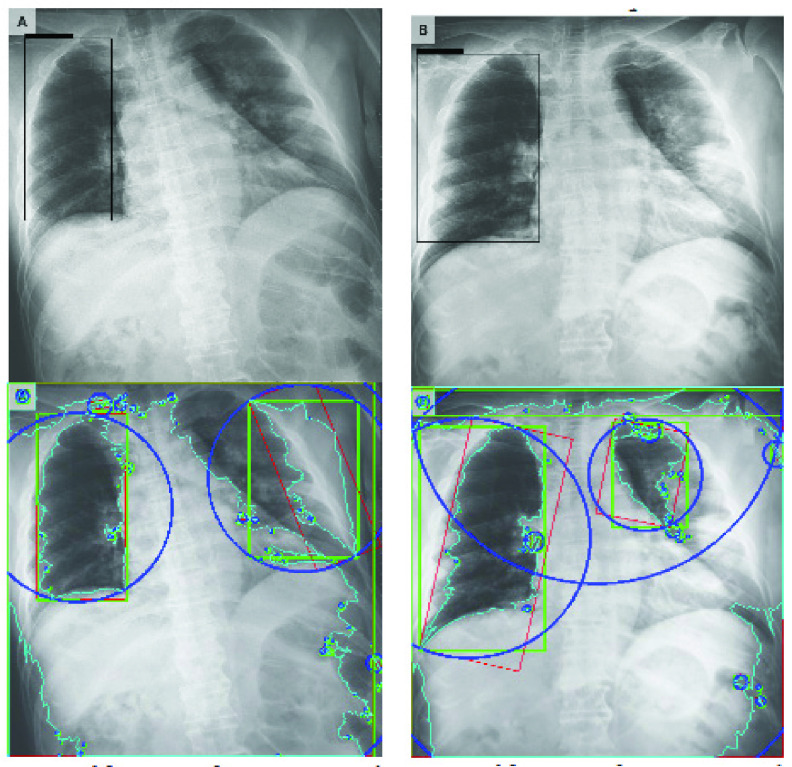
Samples of images and their respective findings with expert annotation as extracted from the NIH Chest X-Ray dataset. The bounding boxes show the suspected regions of COVID-19 in PA view. Below is a capture of the contours in the image samples.
